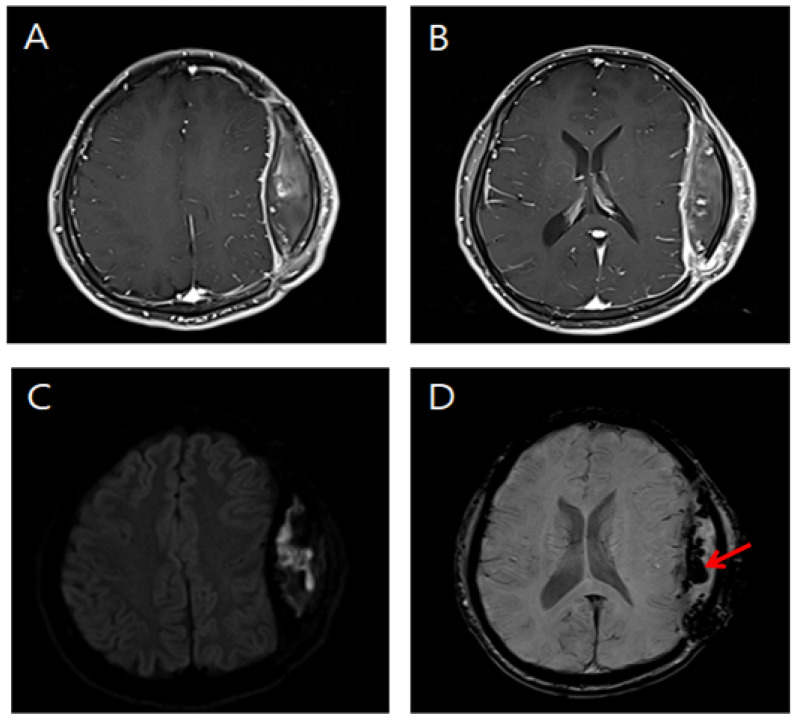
Brain magnetic resonance images of the patient. (A,B) Post-contrast T1-weighted images show rim enhancement of the fluid collection. (C) Diffusion-weighted image of the epidural fluid collection demonstrates restricted diffusion. (D) Susceptibility-weighted image of the epidural fluid collection reveals multiple punctate hypointensities secondary to hemorrhage (arrow). Brain magnetic resonance imaging showed a mass effect caused by a hemorrhagic component and epidural abscess (Figure 4).
